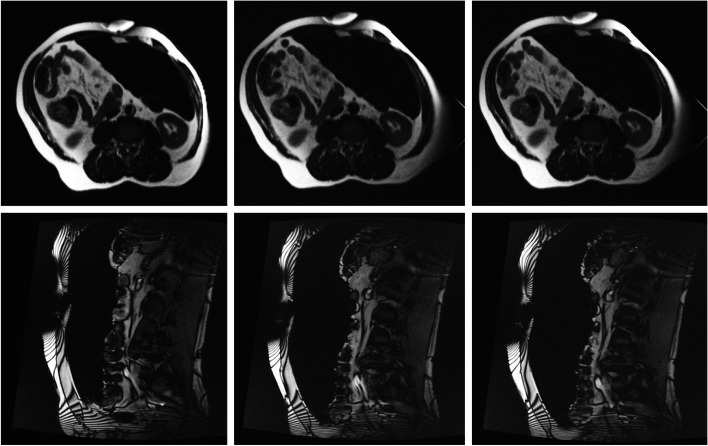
Axial and sagittal MRI slides at different levels of NMB (patient 10). From left to right: T1 (no NMB), T2 (moderate NMB), T3 (deep NMB)
